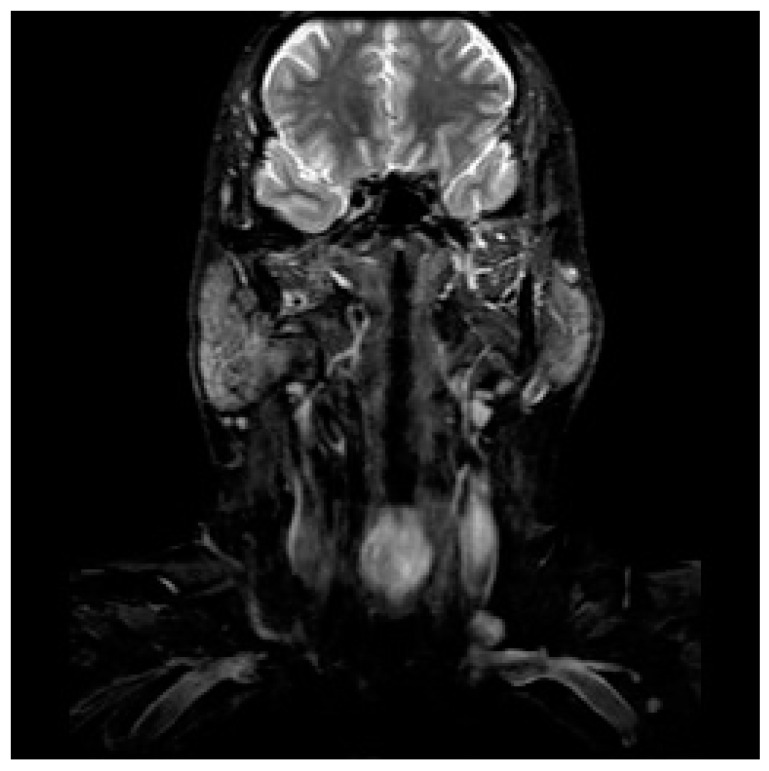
MRI scan of a patients with a laryngeal adenoid cystic carcinoma.
